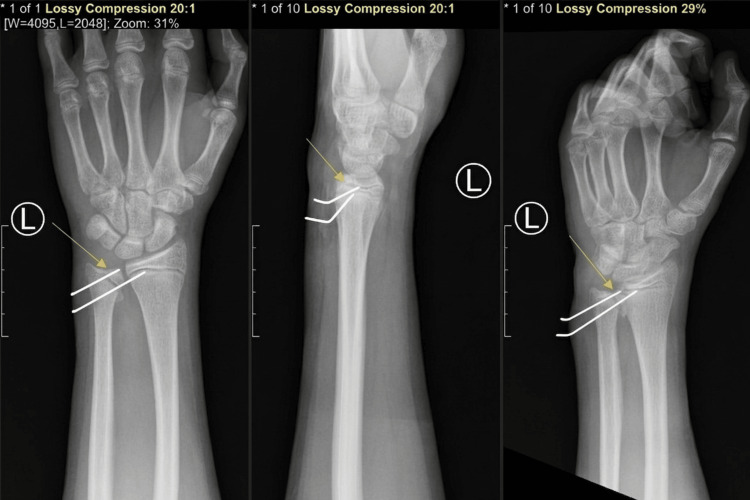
Radiographs obtained immediately after the surgery Postoperative radiographs of the left wrist anteroposterior/posteroanterior (AP/PA; left panel), lateral (middle panel), and oblique (right panel), demonstrating Kirschner wire fixation of the distal ulna (Day one).
